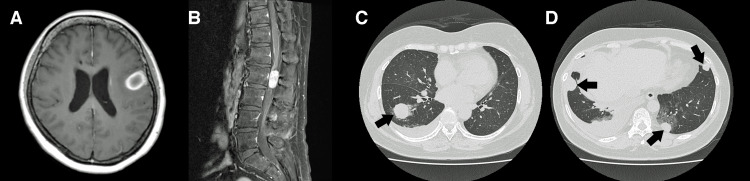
MRI and Computed Tomography Six Months After Initial Treatment A and B: Gadolinium-enhanced MRI six months after initial treatment demonstrating dissemination in the left frontal lobe and the lumbar spinal cord. C and D: Computed tomography six months after initial treatment revealing multiple lung metastases (arrows). MRI: magnetic resonance imaging
